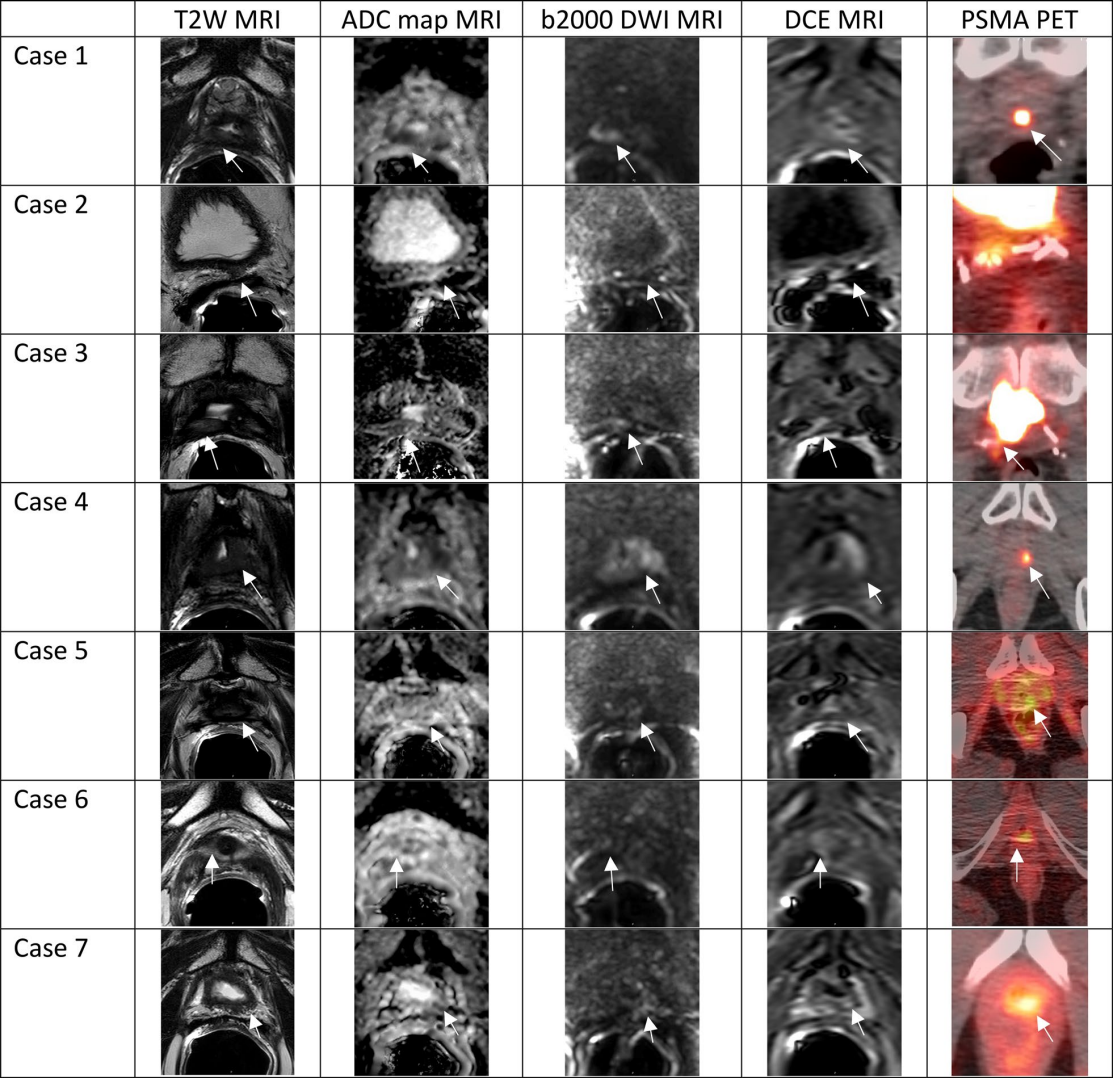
Axial MRI and ^18^F-PSMA PET/CT images of each of the seven patients with isolated local recurrence. White arrows point to areas of interest
